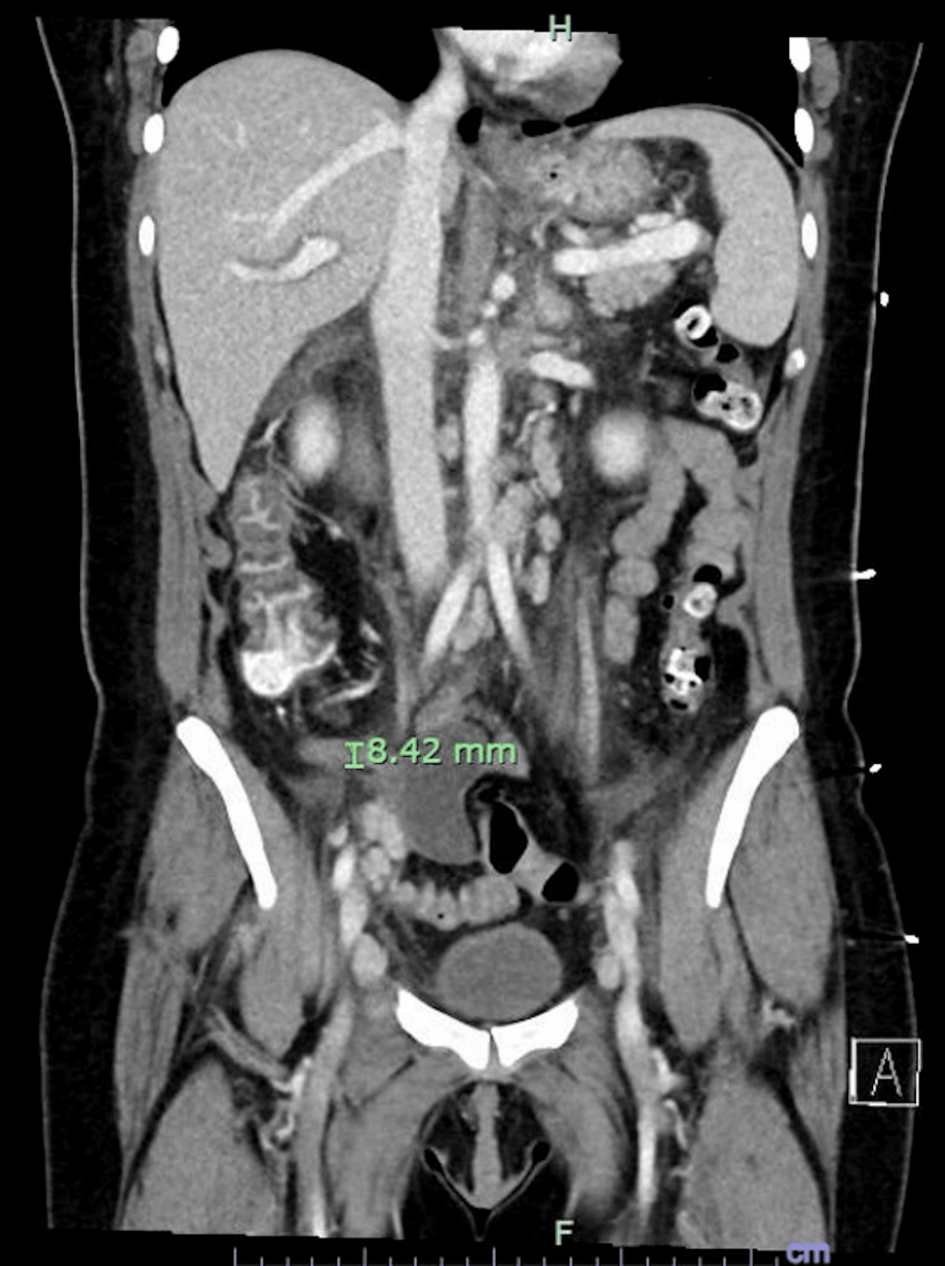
CT of the abdomen and pelvis with contrast, showing appendiceal thickening (marked, 8.42 mm) and diffuse lymphadenopathy.
